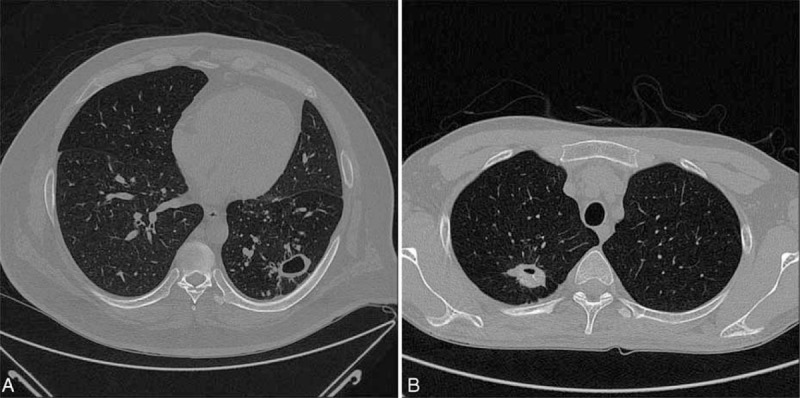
The chest computed tomography scans of our patients with multidrug-resistant pulmonary tuberculosis undergoing video-assisted thoracoscopic surgery wedge resection for (A) left lower lobe and (B) right upper lobe cavities.
